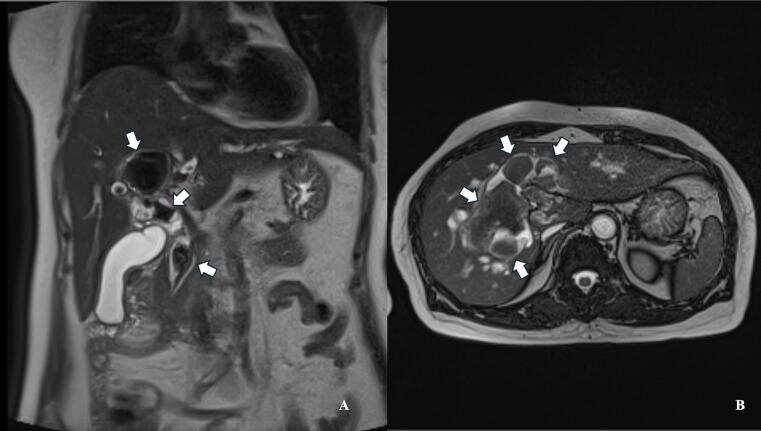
Magnetic resonance imaging of hepatolithiasis. (A) Coronal and (B) axial T2-weighted MRI images demonstrating multiple hypointense filling defects (white arrows) within dilated intrahepatic ducts. The largest stone, measuring 3.3 × 5.6 cm, is visible in the right intrahepatic duct. T2-weighted images provide excellent contrast between the hyperintense bile and hypointense stones.
